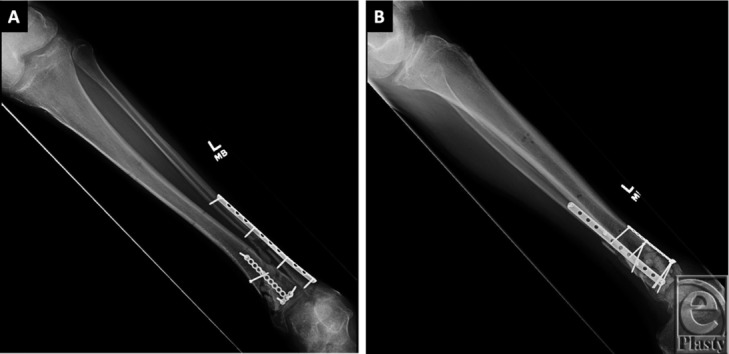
(a, b) Anteroposterior and lateral radiographs of left tibia and fibula showing bridging plates with screws transfixing visible fractures of the distal tibia and fibula. There are mildly displaced butterfly fracture fragments of both bones. There is no radiographic evidence of bony bridging of the fracture fragments.
